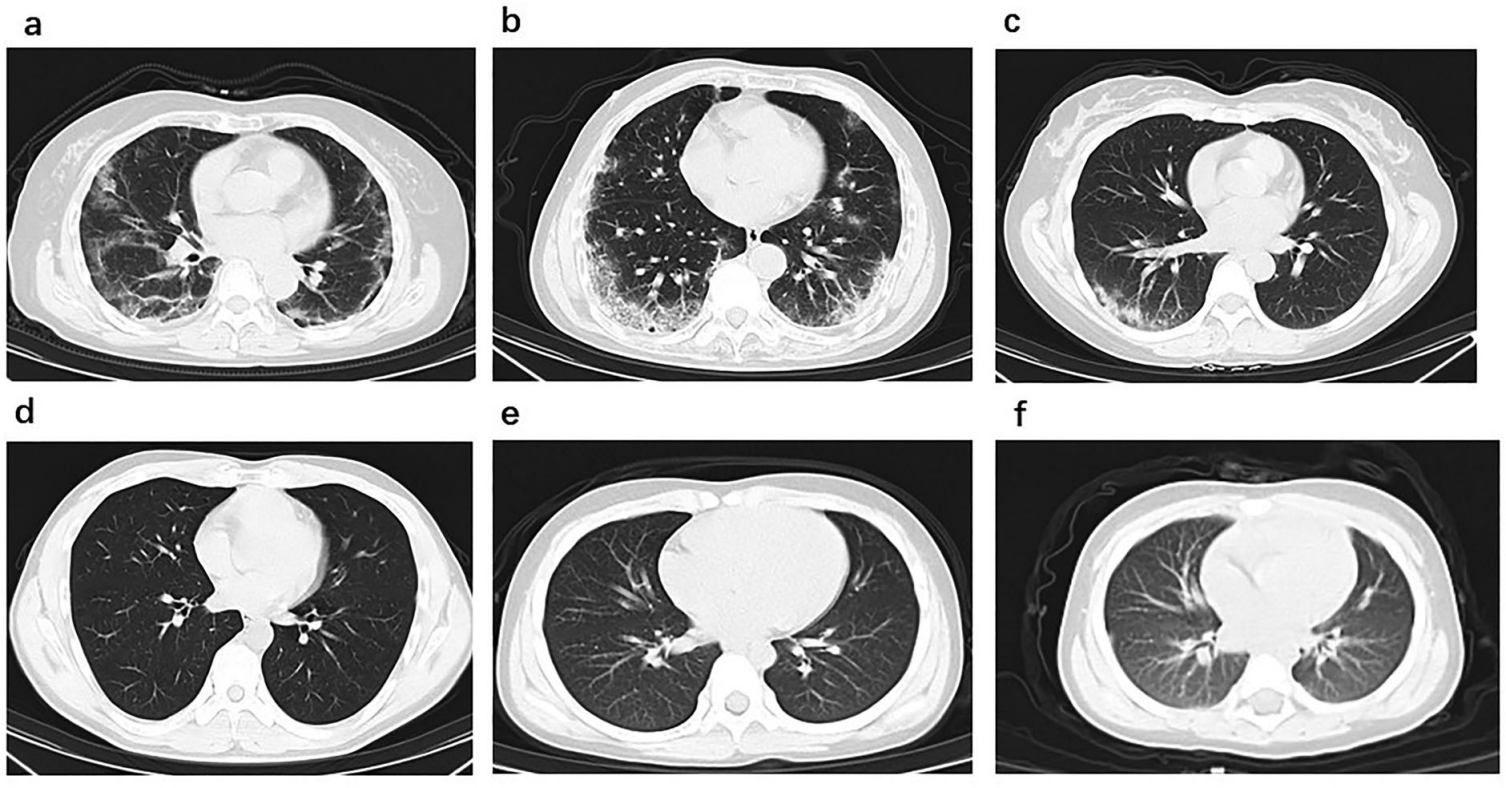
Chest CT images. (a) Transverse chest CT images from Case 1 showing bilateral ground-glass opacity, subsegmental areas of consolidation and subpleural line. (b) Transverse chest CT images from Case 2 showing peripheral pulmonary parenchymal ground-glass and consolidative pulmonary opacities. (c) Transverse chest CT images from Case 3 showing subsegmental areas of ground-glass opacity and consolidation. Transverse chest CT images from Case 4 (d), Case 5 (e) and Case 6 (f) were normal.
